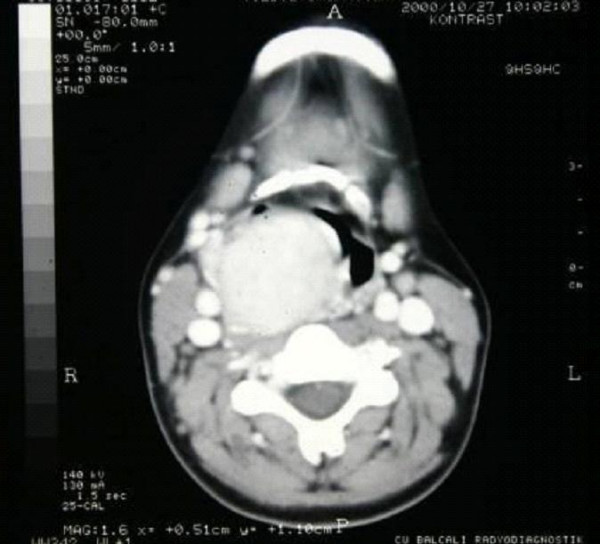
CT scan demonstrated a large, contrast enhancing soft tissue lesion pushing the larynx medially.
